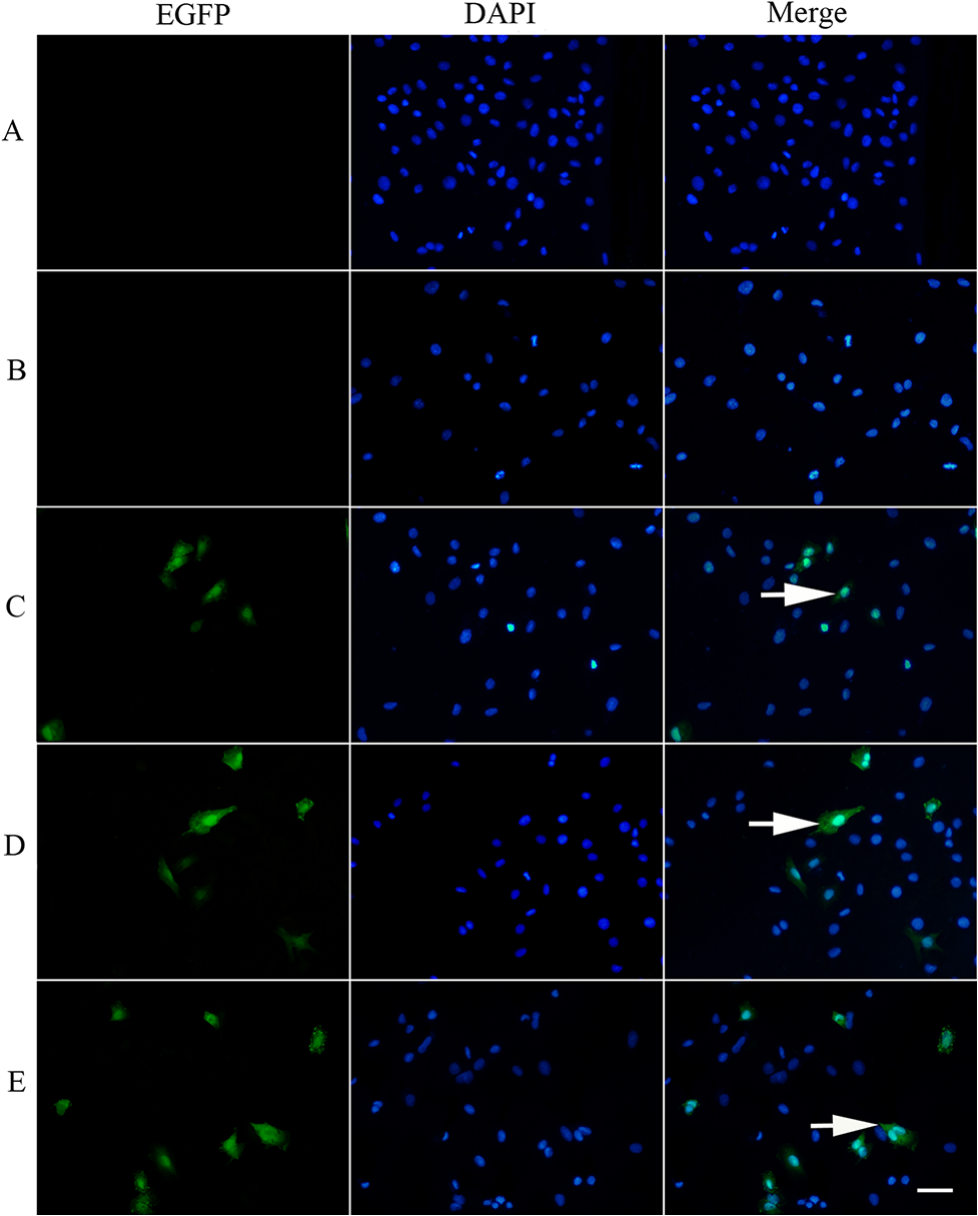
EGFP expression in H9C2 Cells of Group A-E at 48 h post-transfection (200×). (A): untreated group; (B): treated with pEGFP-N plasmid DNA; (C): treated with PEI/DNA complexes; (D): treated with PEI/DNA complexes with ultrasonic exposure; (E): treated with PEI/DNA complexes plus PFPMS with ultrasonic exposure.
